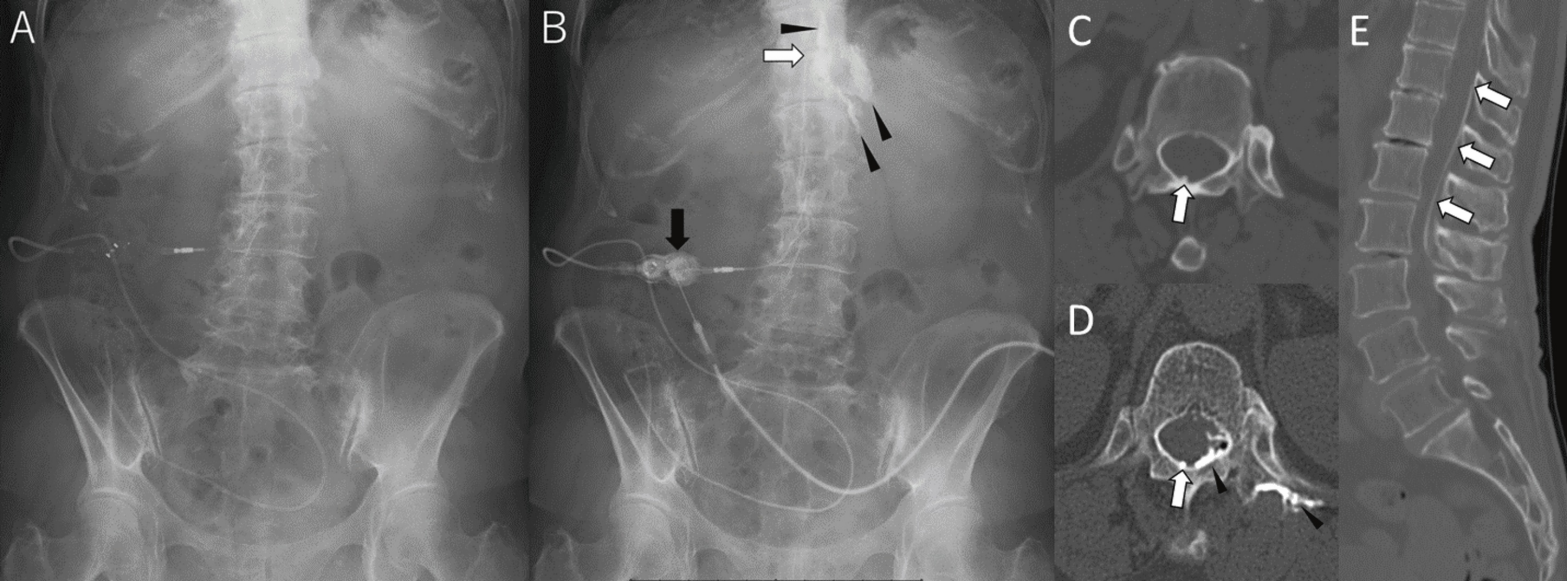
Shuntography findings LP: lumboperitoneal; CT: computed tomography (A) The LP shunt appears to be normally positioned on plain radiography. (B) After puncturing the reservoir with a 23 G needle and injecting contrast medium (black arrow), the contrast travels through both the lumbar and peritoneal catheters but fails to diffuse into the spinal canal. Instead, localized contrast accumulation is observed along the right T11–T12 nerve roots (arrowhead). (C) Axial CT image at the T12 level shows the catheter tip positioned within the spinal canal but adjacent to its dorsolateral wall. (D) Contrast is distributed along the dorsolateral subdural extra-arachnoid space and nerve roots, without entry into the subarachnoid space. (E) Sagittal CT reconstruction further confirms the dorsolateral position of the catheter tip within the spinal canal
